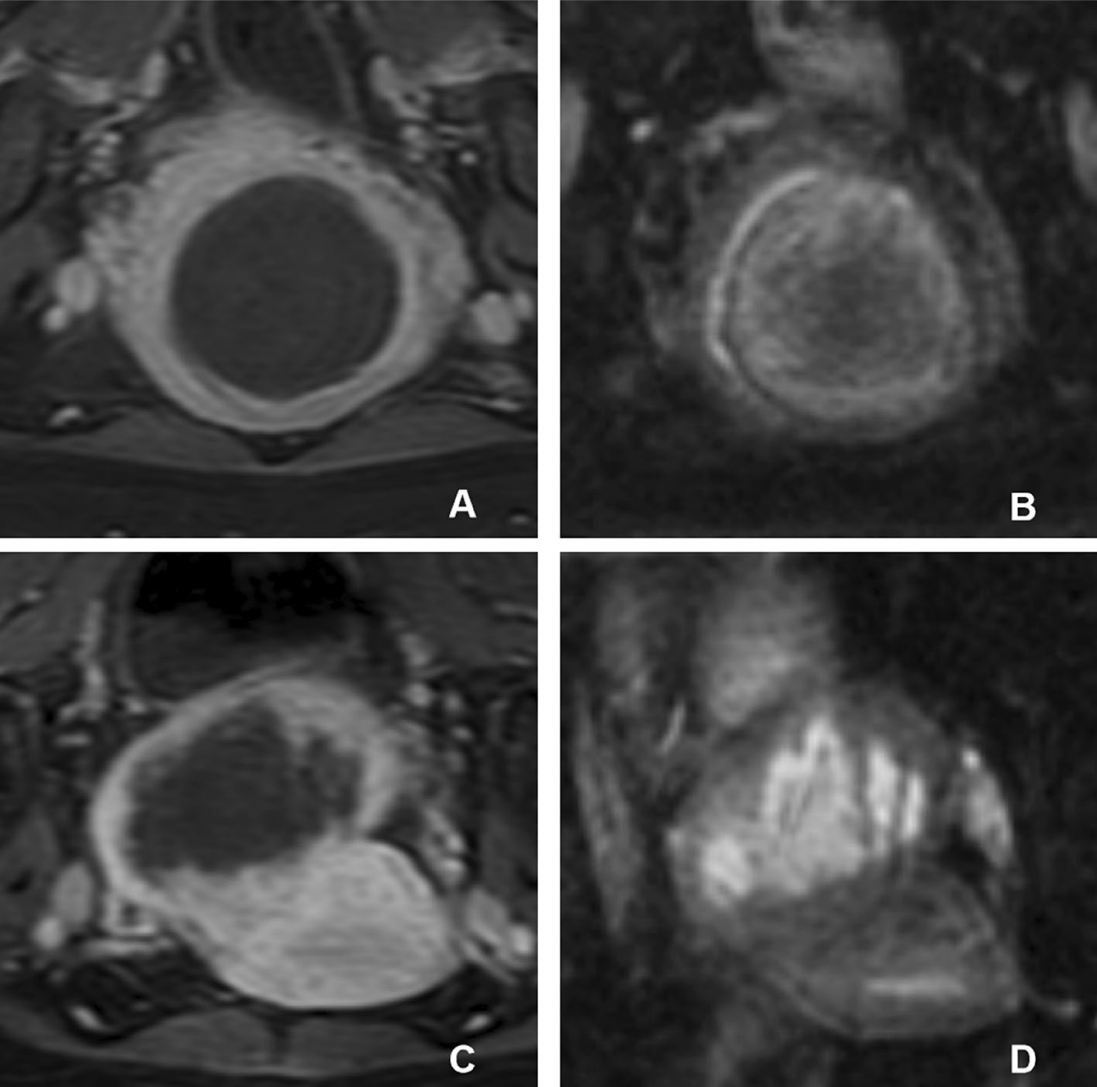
Classification of DWI signal types in ablated necrotic lesions. (A,C) Contrast-enhanced T1-weighted images post-ablation reveal non-enhancing hypointense zones indicative of ablated necrotic uterine leiomyomas. (B,D) Corresponding DWI images post-ablation. (B) The central hypointense lesion with a surrounding hyperintense ring in the same lesion as in (A) is characteristic of type 1, known as the “bull’s eye sign”. (D) The inhomogeneous hyperintense lesion lacking a ring in the same lesion as in (C) is classified as type 2, referred to as the “bright patch sign”. *DWI* diffusion-weighted imaging.
